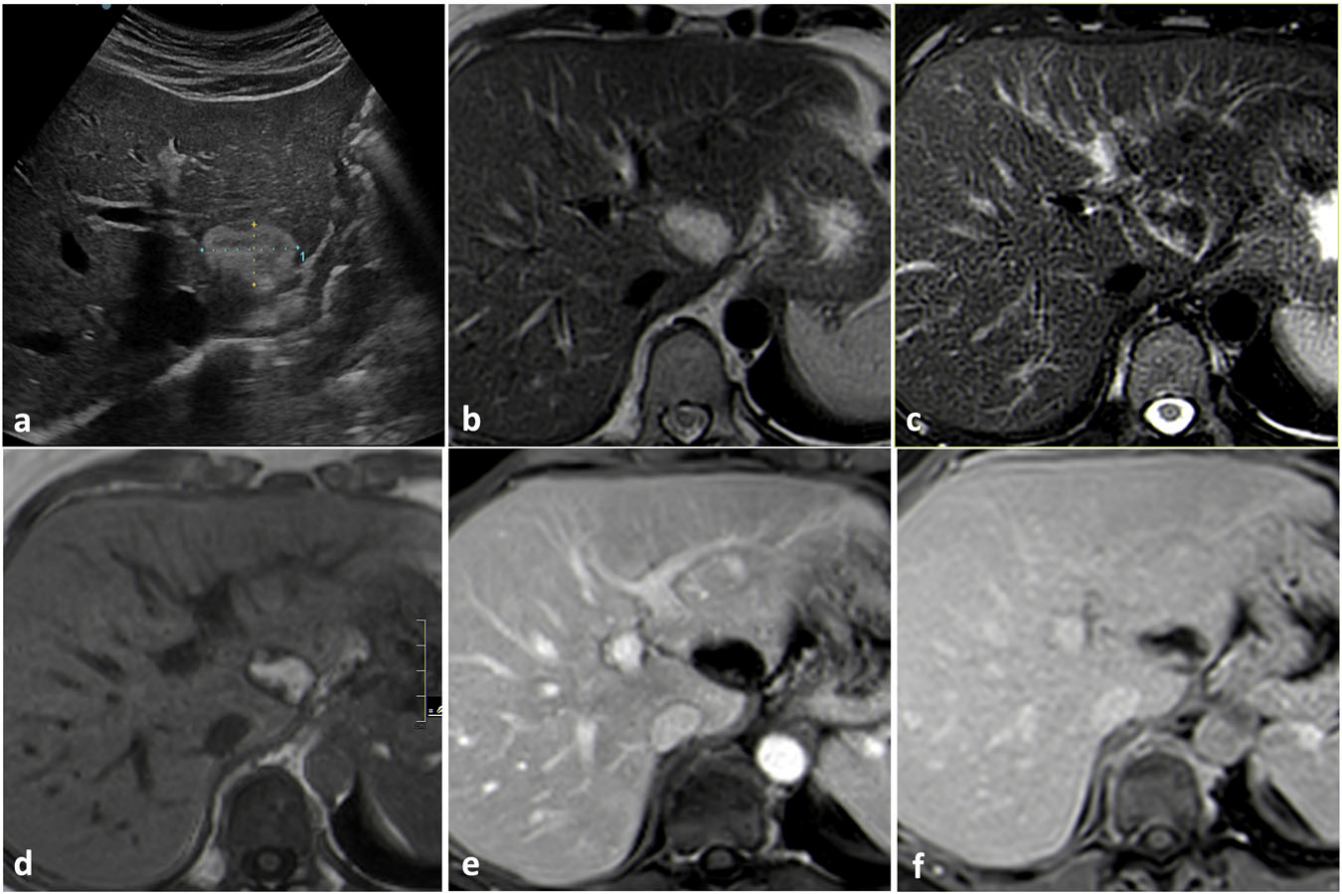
Angiomyolipoma. On B-mode US (a) a heterogeneous hyperechoic lesion is appreciable. The lesion appears markedly hyperintense on T2-weighted images (b) becoming prevalently hypointense after fat saturation (c) because of the macroscopic fatty content. On T1-weighted images (d) the lesion appears heterogeneously hyperintense; the peripheral components resemble a globular enhancement pattern during arterial (e) and portal venous (f) phases
